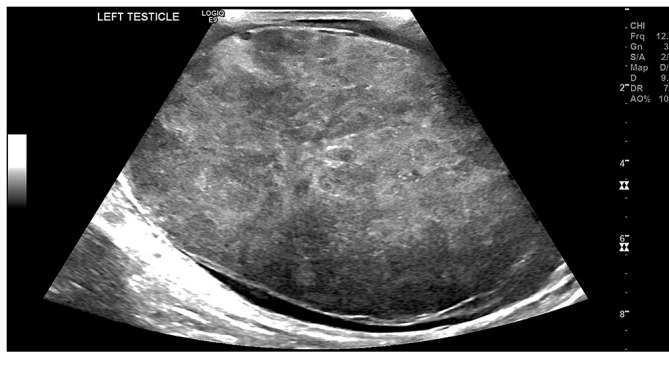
B-Mode ultrasound image of the left testicle which shows a diffuse, heterogenous echotexture.
